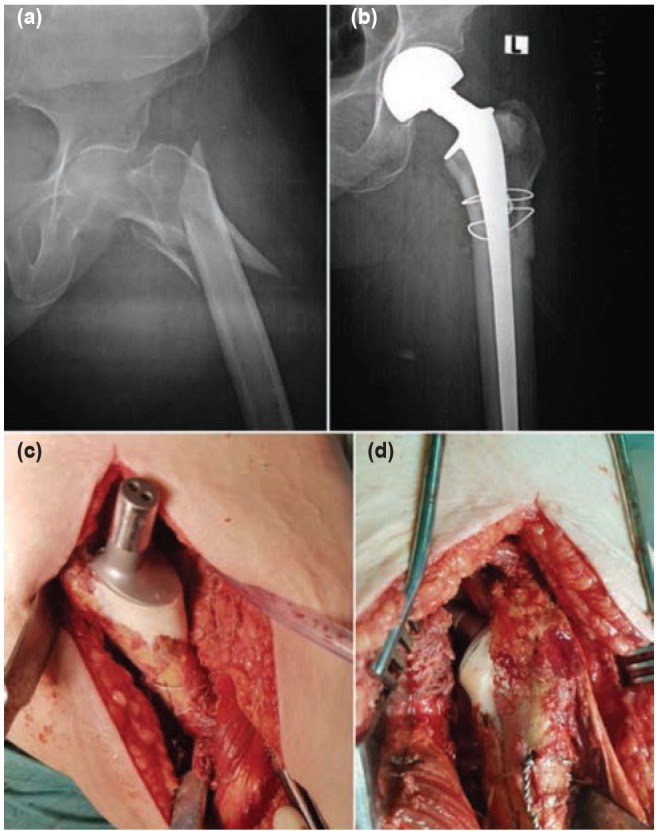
Unstable reverse oblique fracture with (a) Pre- and (b) Post-operative radiographs and intraoperative images showing the reconstruction of the greater trochanter and the cemented stem (c) Before and (d) After reduction.
